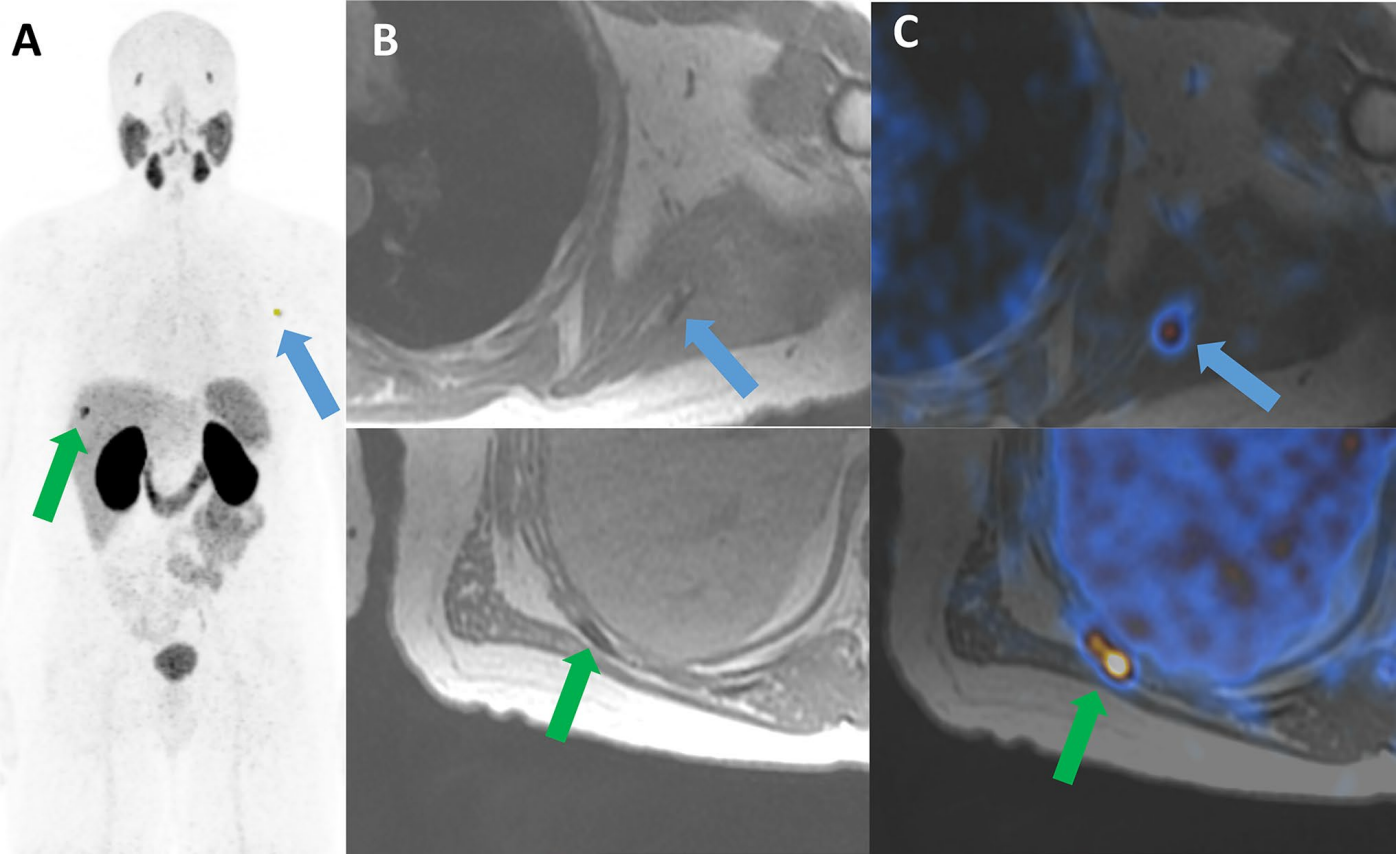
Patient with oligometastatic prostate cancer. 71-year-old patient initially following laparoscopic prostatectomy for pT3b pN0 cM0, Gleason 4 + 5 PCa. Restaging using ^68^Ga-PSMA-11 PET/MRI is performed due to a rising PSA to 0.8 ng/ml under established ADT using Leuporelin. a Coronal MIP of ^68^Ga-PSMA-11 with corresponding axial slides of fat weighted LAVA-DIXON slides b over the left scapular (blue arrow) and right rip (green arrow) metastases, and fused PET/MR c images showing the intense uptake in both lesions
